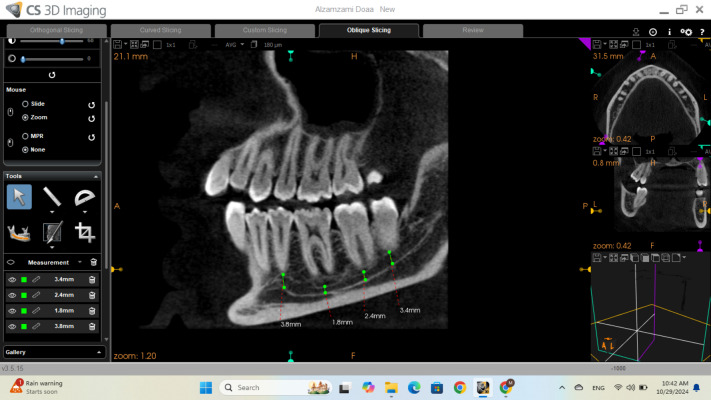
Sagittal view of CBCT showing the measurement of the inferior alveolar diameter.
